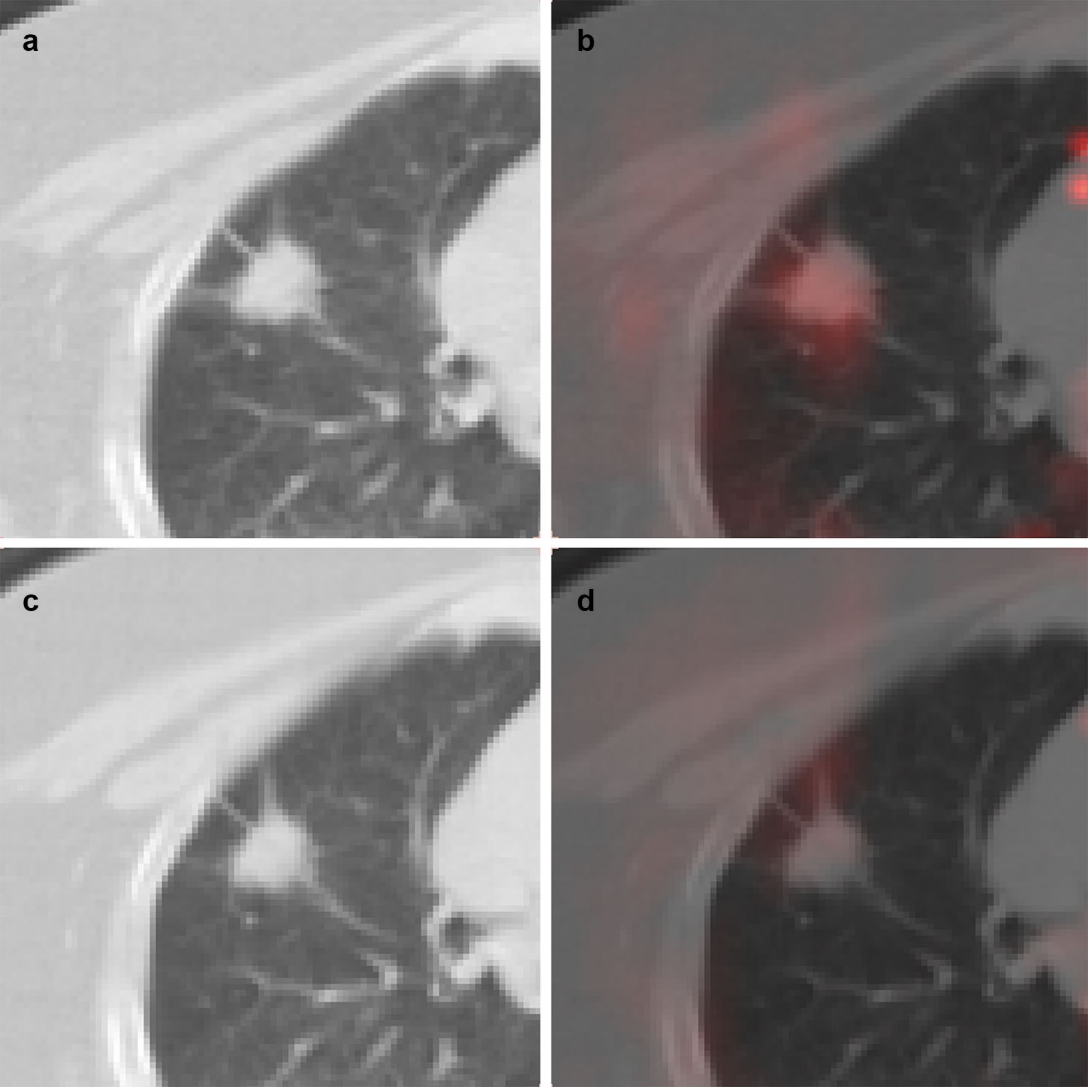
^15^O-water positron emission tomography of a 67-year-old woman with adenocarcinoma treated with bevacizumab (patient no. 2). (a,b) CT and fusion image of CT and parametric image of blood flow at baseline. (c,d) CT and fusion image after chemotherapy. CT depicted a 2.6-cm nodule in the right lung. Tumor blood flow was markedly reduced, from 0.256 mL/cm^3^/min to 0.059 mL/cm^3^/min (blood flow ratio 0.23). Three months later the tumor exhibited progression.
